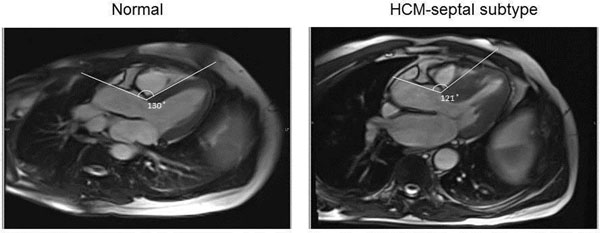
Patients with septal variant of hypertrophic cardiomyopathy exhibit more acute angulated septum than normal controls. The angulated septal angle, measured as the angle between the right septal surface and anterior aortic wall, is shown in the MRI of a normal control (left) and a patient with septal variant of HCM (right).
